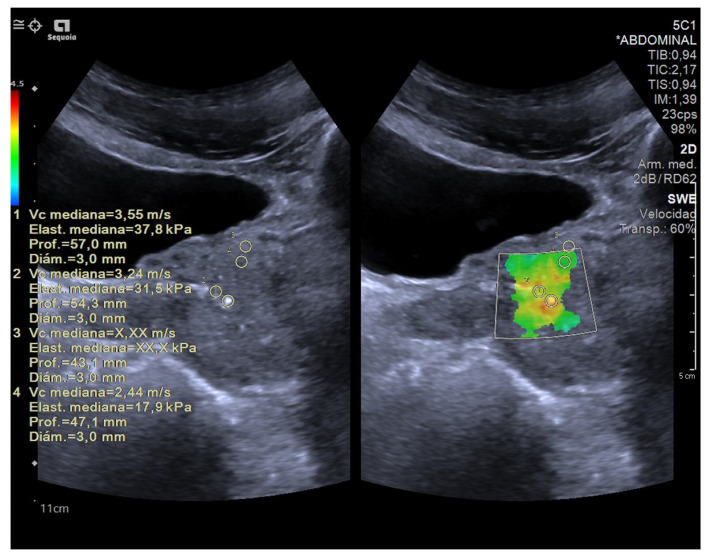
41 year-old patient with elevated PSA without other clinical data of interest. Prostate ultrasound image with a suprapubic approach performed using a SIEMENS Sequoia ultrasound device with a Convex 5C1 probe. The elasticities of the tissues observed in the ROIs were 37.8 kPa in ROI 1; 31.5 kPa in ROI 2 and 17.9 kPa in ROI 4; ruling out the absence of malignant disease. Adjacent to the prostate, a hypoechoic area was identified, corresponding to an abscess, with the diagnosis being prostatitis. The absence of data indicating tissue stiffness in ROI 3 may be due to the heterogeneity of the sample.
